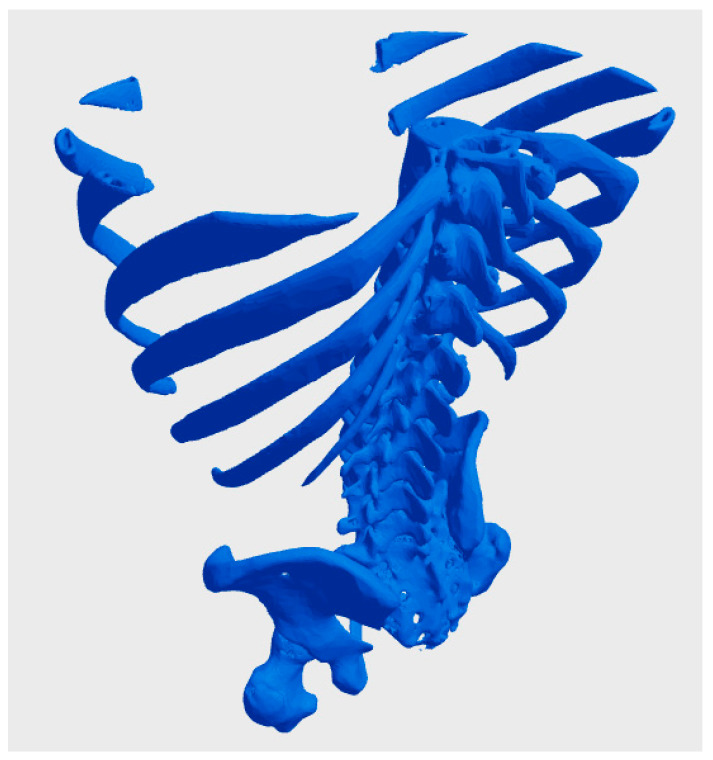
bio-CAD model derived from a real CT scan of a patient’s thorax and pelvis, freely available on the Thingiverse website under a Creative Commons license [61].
